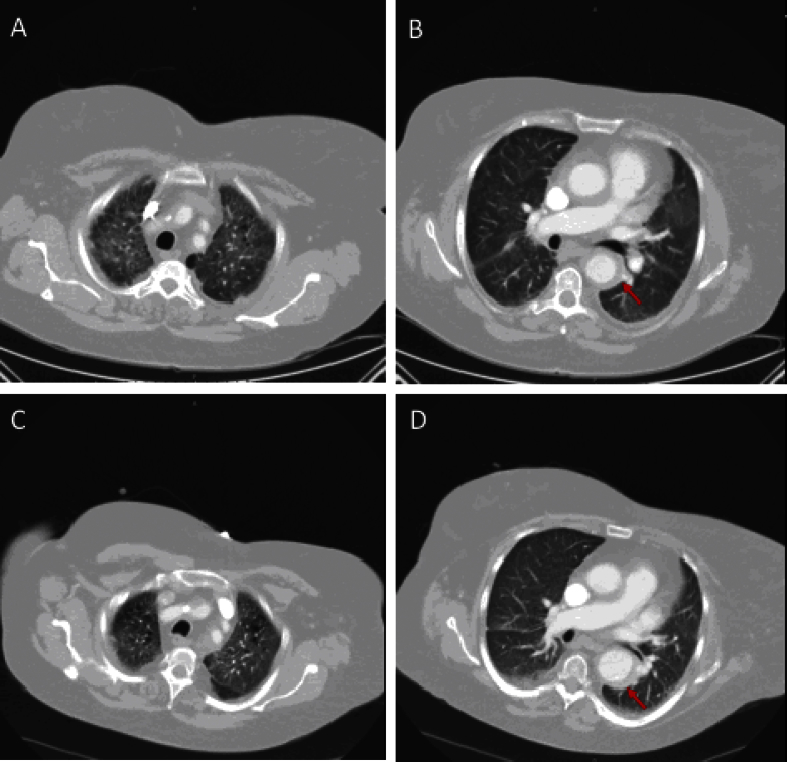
Panel A and B. Initial CT demonstrating typical peri-aortic thickening, ground-glass opacities as well as the presence of cysts. Panel C and D. Follow-up CT chest shows stable disease following 6 months of treatment with vemurafenib.
